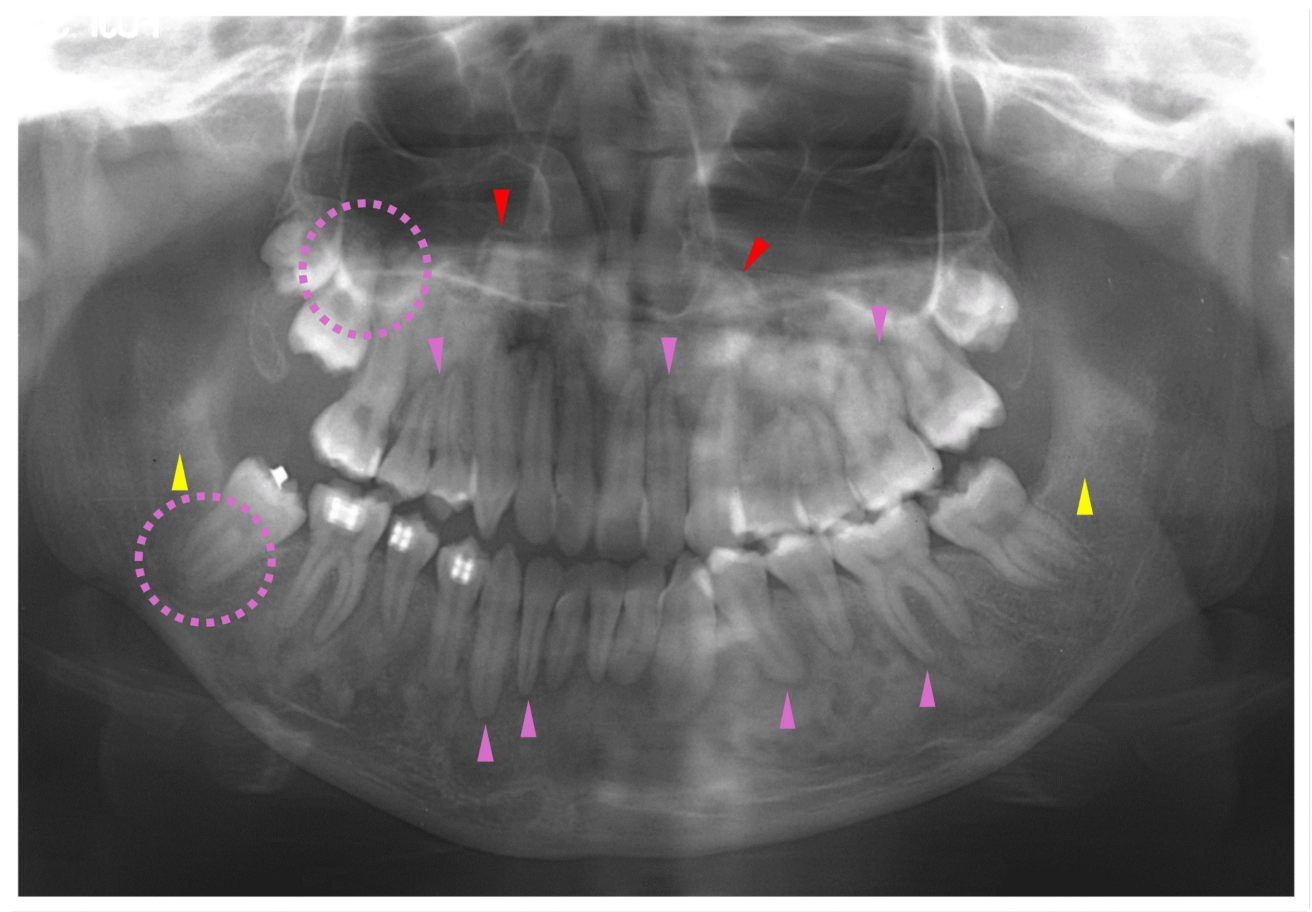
Maxillofacial radiograph at age 14 Yellow arrowheads indicate normal healings of the mandibular bone sites after the impacted wisdom teeth extraction. Purple dotted circles indicate bony adhesions (ankylosis) in the right upper and lower second molars. Purple arrowheads indicate widening of the periodontal ligament spaces. Red arrowheads indicate stenosis of the maxillary sinus
